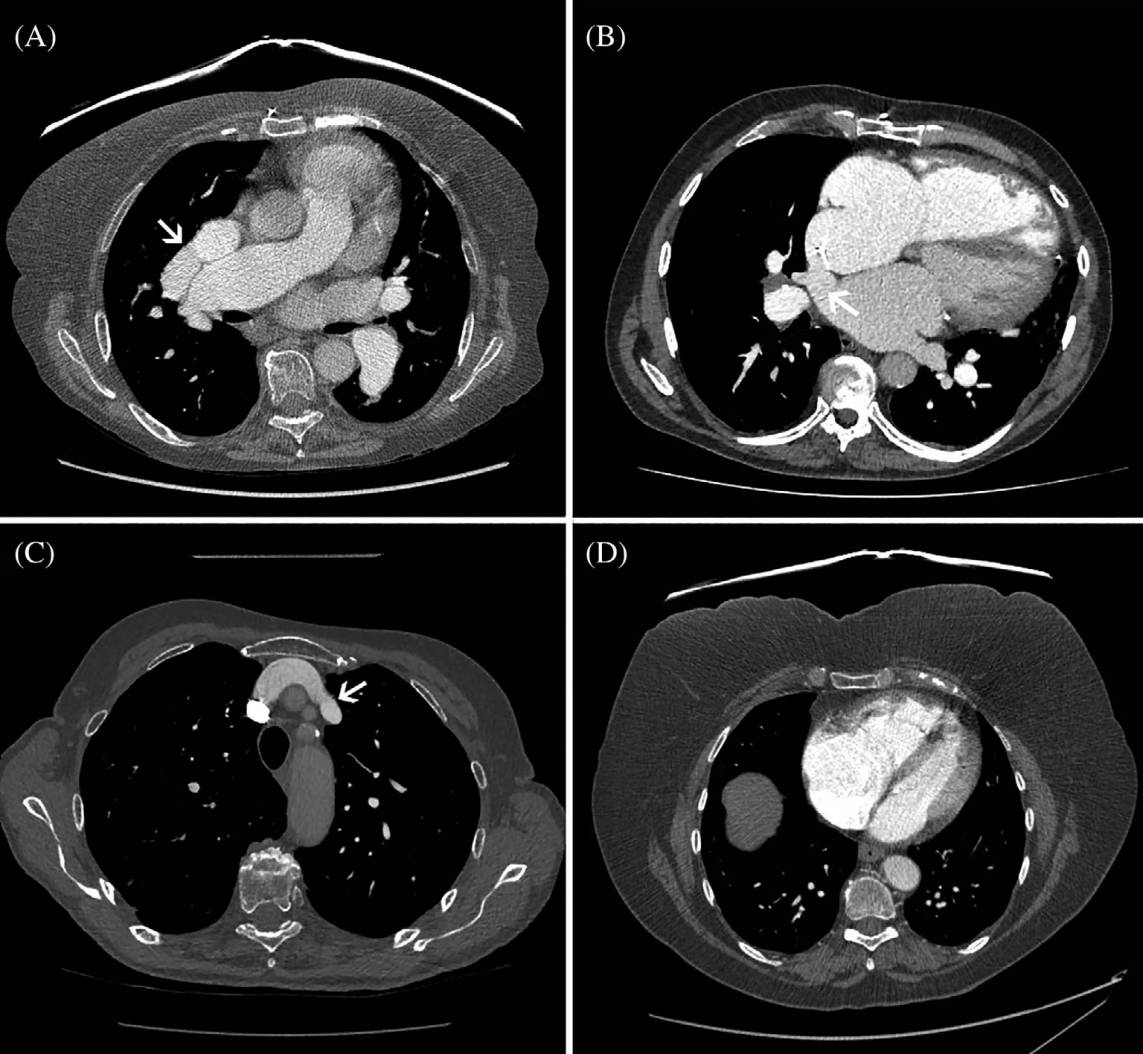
Contrast‐enhanced CT scans. (A) Anomalous drainage of right superior vein into SVC (arrow). (B) SV‐ASD (arrow). (C) Anomalous drainage of left superior vein into brachiocephalic vein (arrow). (D) Enlargement of anteriorly located right heart chambers in a patient with PAPVD. CT, computed tomography; PAPVD, partial anomalous pulmonary venous drainage; SV‐ASD, sinus venosus atrial septal defect; SVC, superior vena cava.
